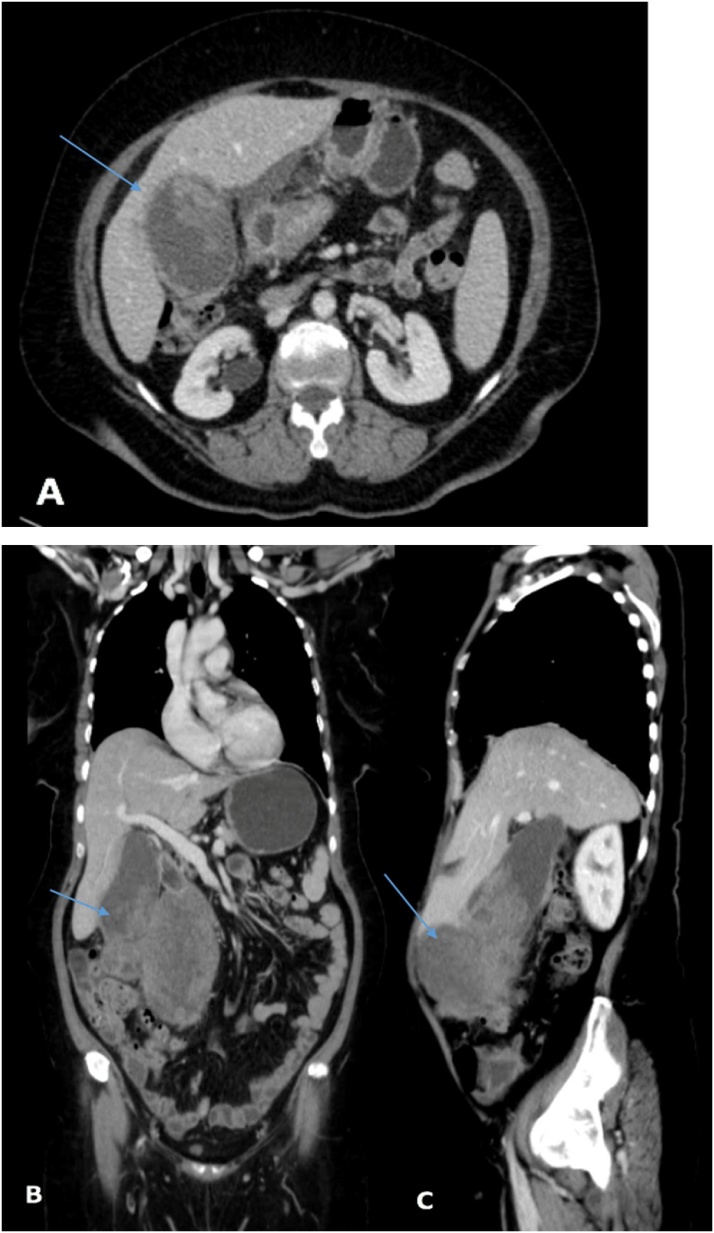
A: Computed tomography scans of the chest and the abdomen revealing a distended gallbladder fundus with intraluminal tumour and huge exophytic mass measuring approximately 13.6 × 12 × 9.5 cm. B: Coronal view of the computed tomography scans of the chest and abdomen revealing a large gallbladder mass; medially the lesion is displacing the distal stomach at the level of pylorus, and the mass protruding downward is displacing the transverse colon inferiorly. Few small portal lymph nodes are observed. C: Sagittal view of the same image.
